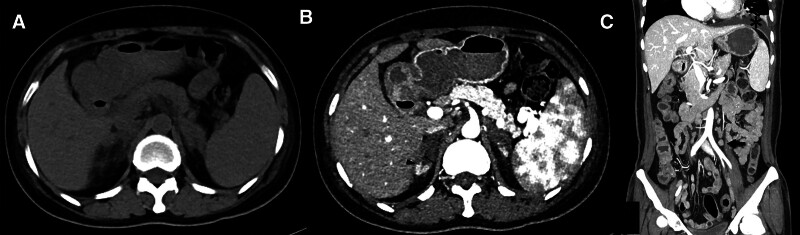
CT features of non-hemorrhagic adrenal infarction. (A) Non-contrast CT scan reveals the thickening adrenal gland with surrounding stranding. (B) Enhanced CT shows the poor enhancement of lesion, “cutoff sign” (arrowhead) and “capsular sign” (arrow). (C) Coronal enhanced CT image demonstrates the enlargement of para-aortic lymph nodes (arrow) and pleural effusion (asterisk). CT = computed tomography.
